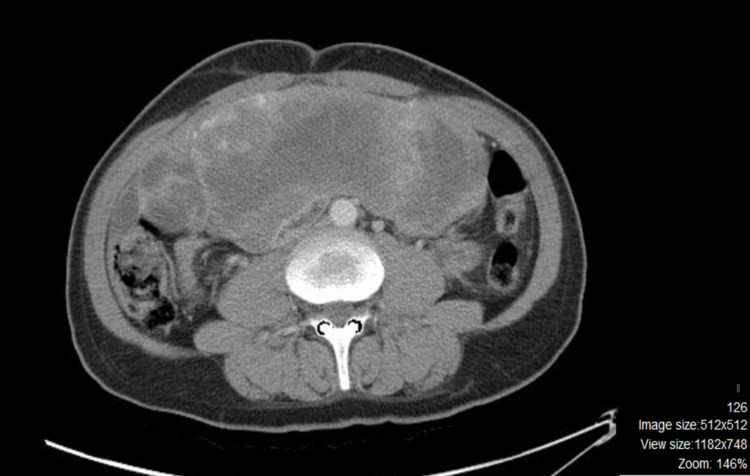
Abdominal computed tomography (CT) imaging after six months of treatment with Imatinib revealing a decrease in the size of the tumor (25x14x22 cm) (axial section).
